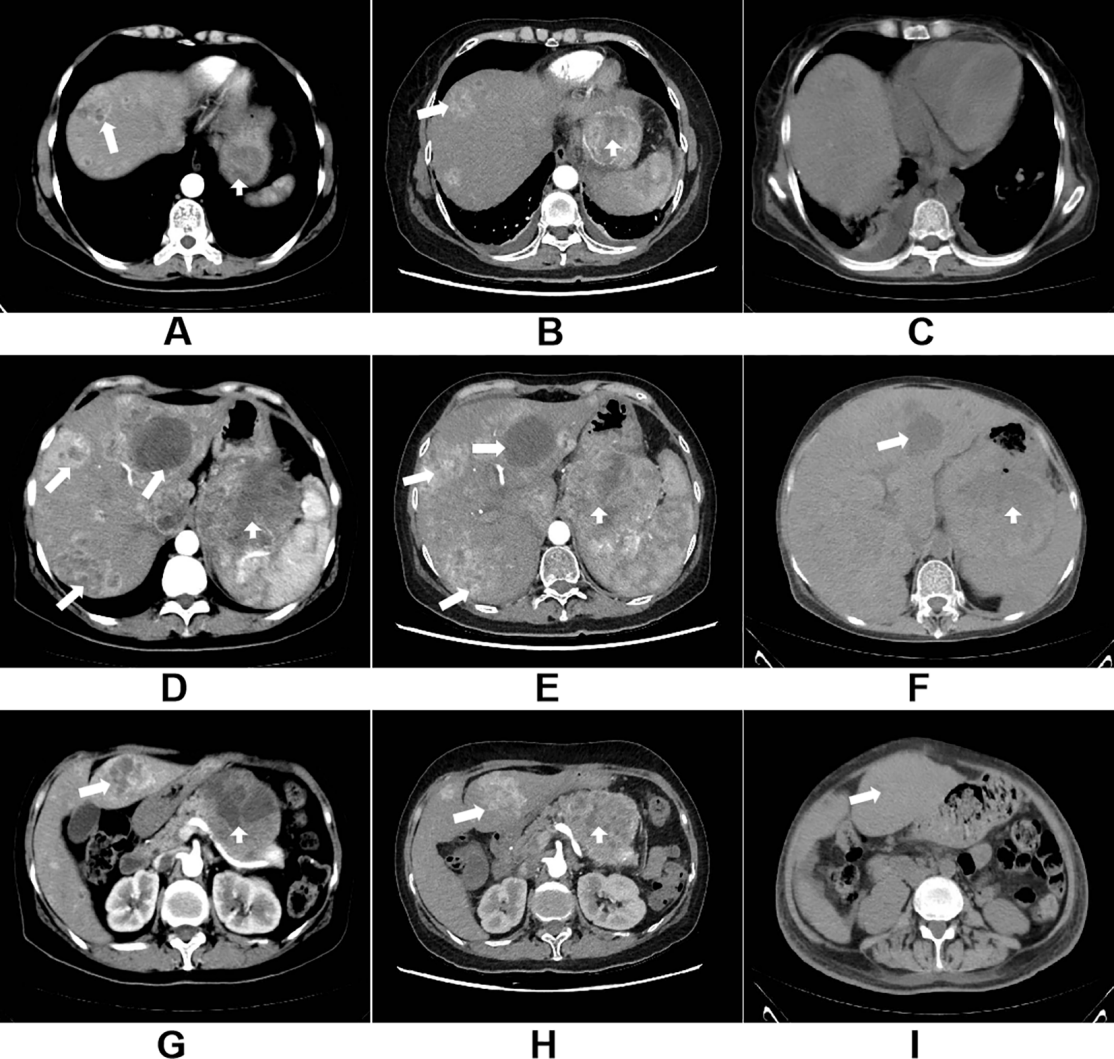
(A, D, G) The contrast-enhanced CT scan of the thorax and abdomen in January 2024 clearly shows the primary gastric hemangiopericytoma lesion and hepatic metastasis; (B, E, H) The enhanced CT scan of the thorax and abdomen in October 2024 shows marked disease progression, with enlargement of both the primary lesion and metastases, as well as the development of pleural effusion; (C, F, I) The non-contrast CT scan of the chest and abdomen in March 2025 indicates continuing disease progression.
